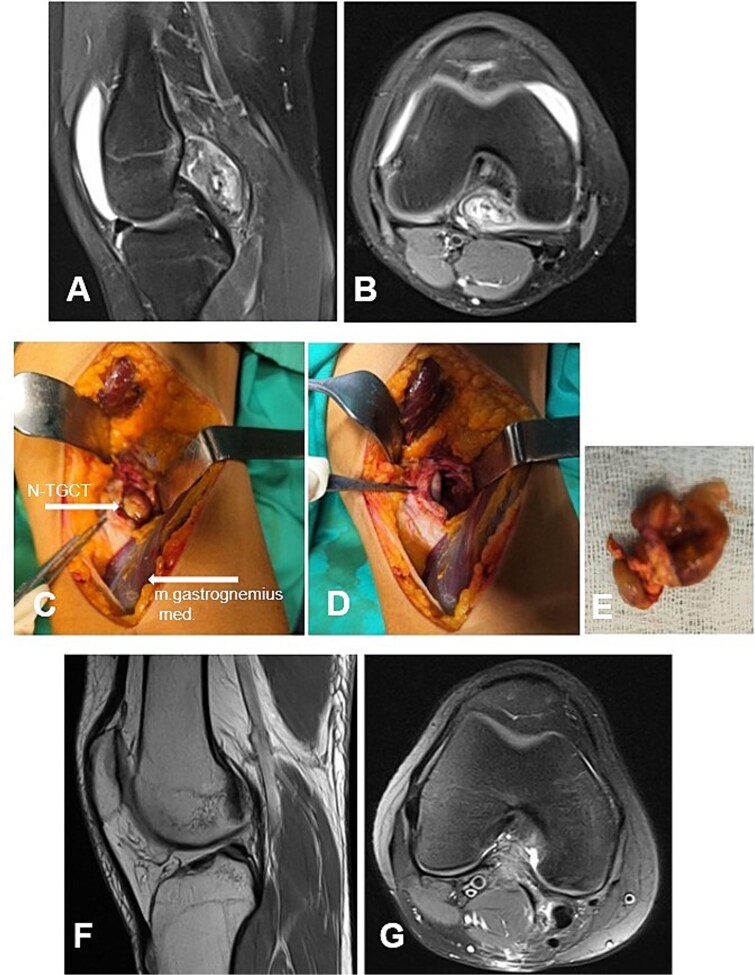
Case 5. (A and B) Preoperative sag and ax MRI TSE PD FS—lobulated edematosus intra-articular neoplasm with a posterior mediocentral localization. (C and D) Posterior synovectomy between m. semitendinosus and m. gastrognemius medialis. (Е) Macroscopic appearance of the N-TGCT measuring 3 × 2 × 2 cm after excision. (F and G) Postoperative 12 m. Sag and Ax MRI TSE PD FS—no evidence of recurrence of the resected neoplasm. PD FS, proton density weighting with fat suppression; TSE, turbo spin echo.
